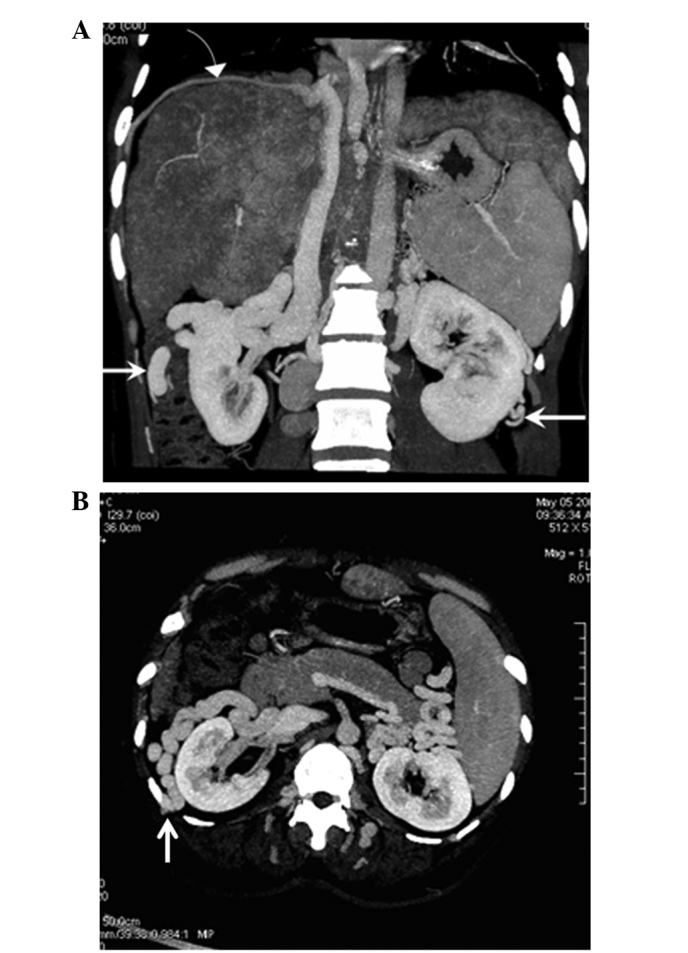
IVC-renal vein-peri-renal vein-superficial epigastric vein collaterals. (A) CTA shows bilateral renal veins communicating with peri-renal veins (straight arrow). The dilated right inferior phrenic vein can also be observed (curved arrow). (B) The transverse section of the CTA shows the distended and tortuous right peri-renal veins anastomosing with the superficial epigastric vein (arrow). IVC, inferior vena cava; CTA, computed tomography angiography.
